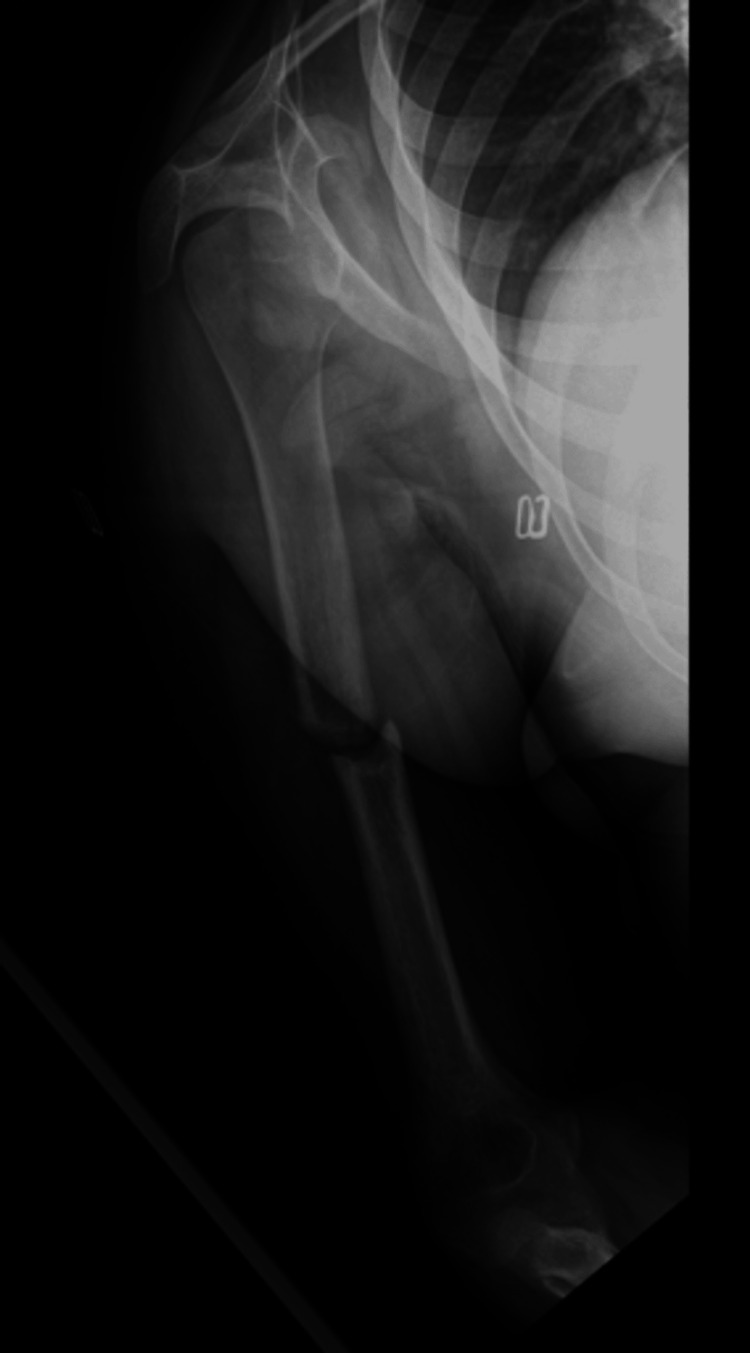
Radiograph of the humerus at five months post-injury. It demonstrates transverse humeral shaft fracture nonunion. No bone formation at the fracture site and no signs of progressive bony changes compared to prior images.
